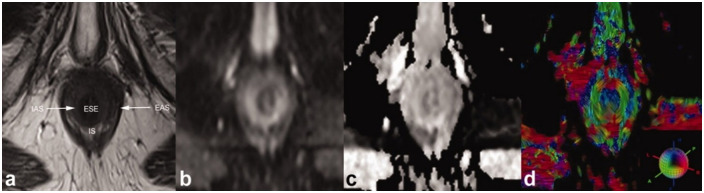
T2-weighted axial image (a) and corresponding diffusion weighted b 0 s/mm2 (b), ADC (c) and color coded FA (d) maps at mid anal canal level. Source: Reprinted from Journal of Magnetic Resonance Imaging (12). Copyright 2025, with permission obtained from Wiley (License No. 6064200283598).
